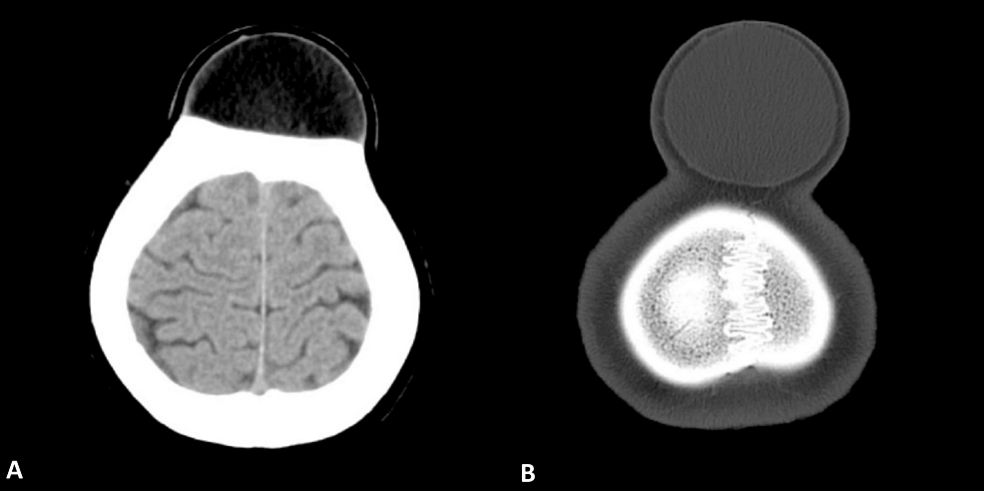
The computed tomography image shows (A) the fat density and (B) the entire lipoma.
